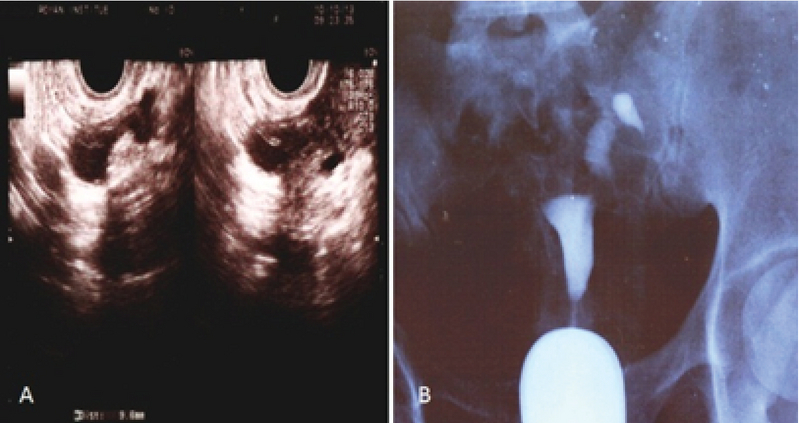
(A) Vaginal ultrasound shows a 10-mm hypoechogenic mass on the right side of the uterus. (B) Hysterosalpingography (HSG) findings in tuberculous salpingitis. HSG shows an occluded bilateral tube.
